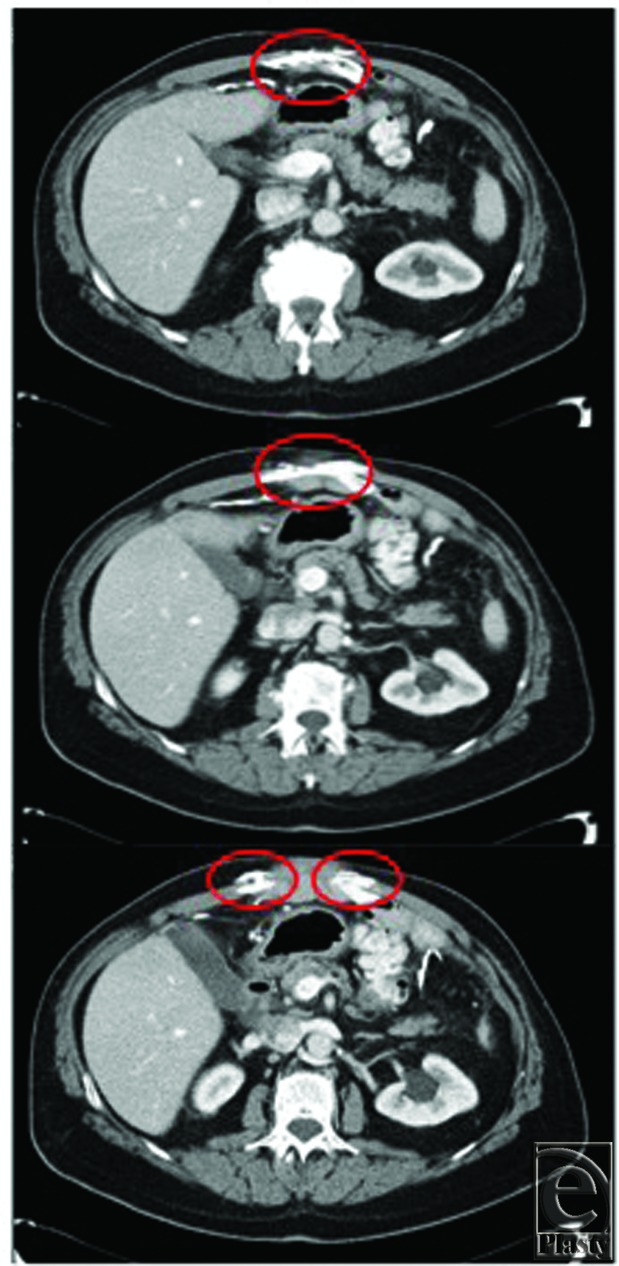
Sequential axial computed tomographic scans of abdominal wall showing cephalad heterotopic ossification invading into rectus muscles and interdigitating between the small bowel.
